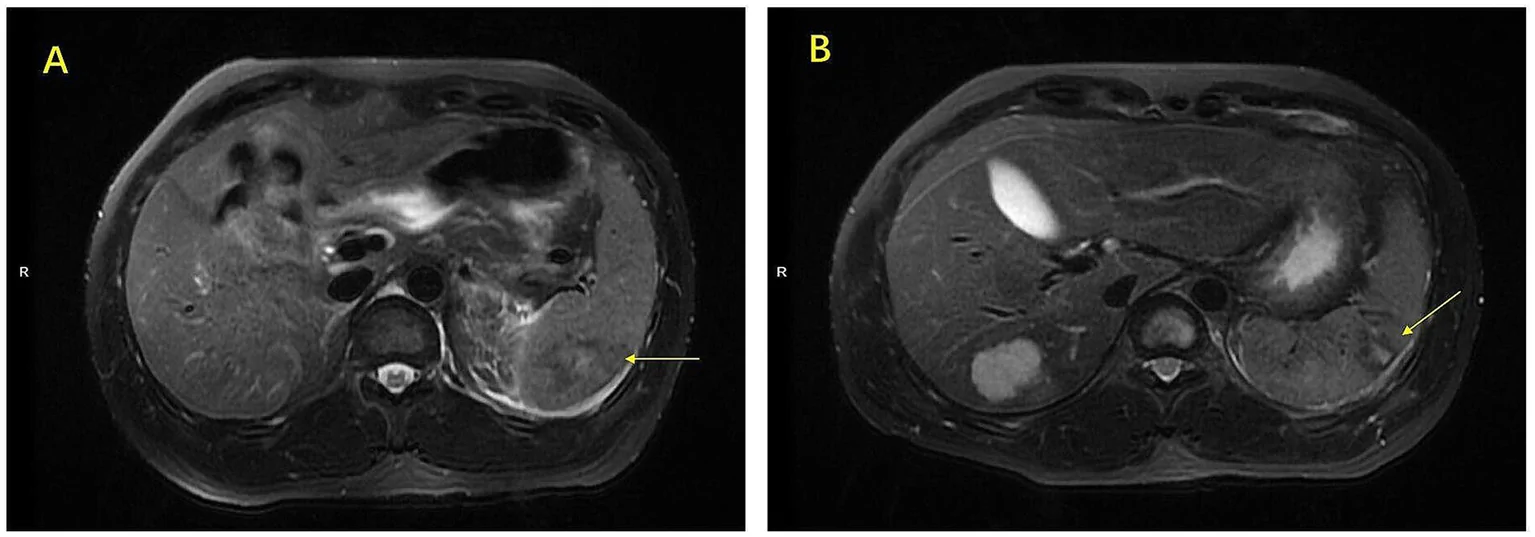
Contrast-enhanced abdominal magnetic resonance imaging of splenic infarction. (A) Axial upper abdominal MR image demonstrates splenomegaly with a peripheral wedge-shaped/patchy abnormal signal in the splenic parenchyma (arrow). (B) Corresponding contrast-enhanced axial image demonstrates a sharply demarcated non-enhancing hypoenhancing splenic lesion (arrow), with a small amount of adjacent perisplenic fluid, consistent with splenic infarction.
